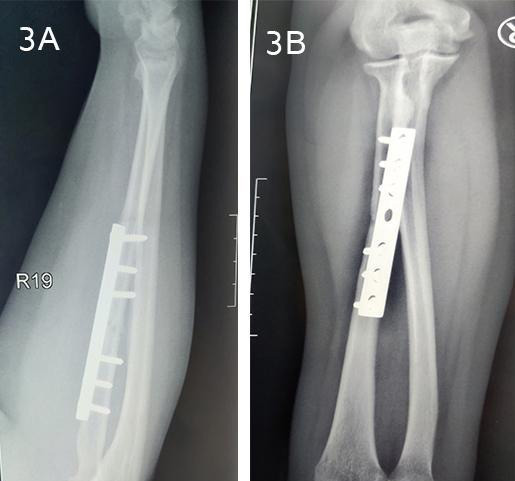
A, B. Plain radiographs at 6 months follow-up (A) Lateral view (B) Anteroposterior view.
